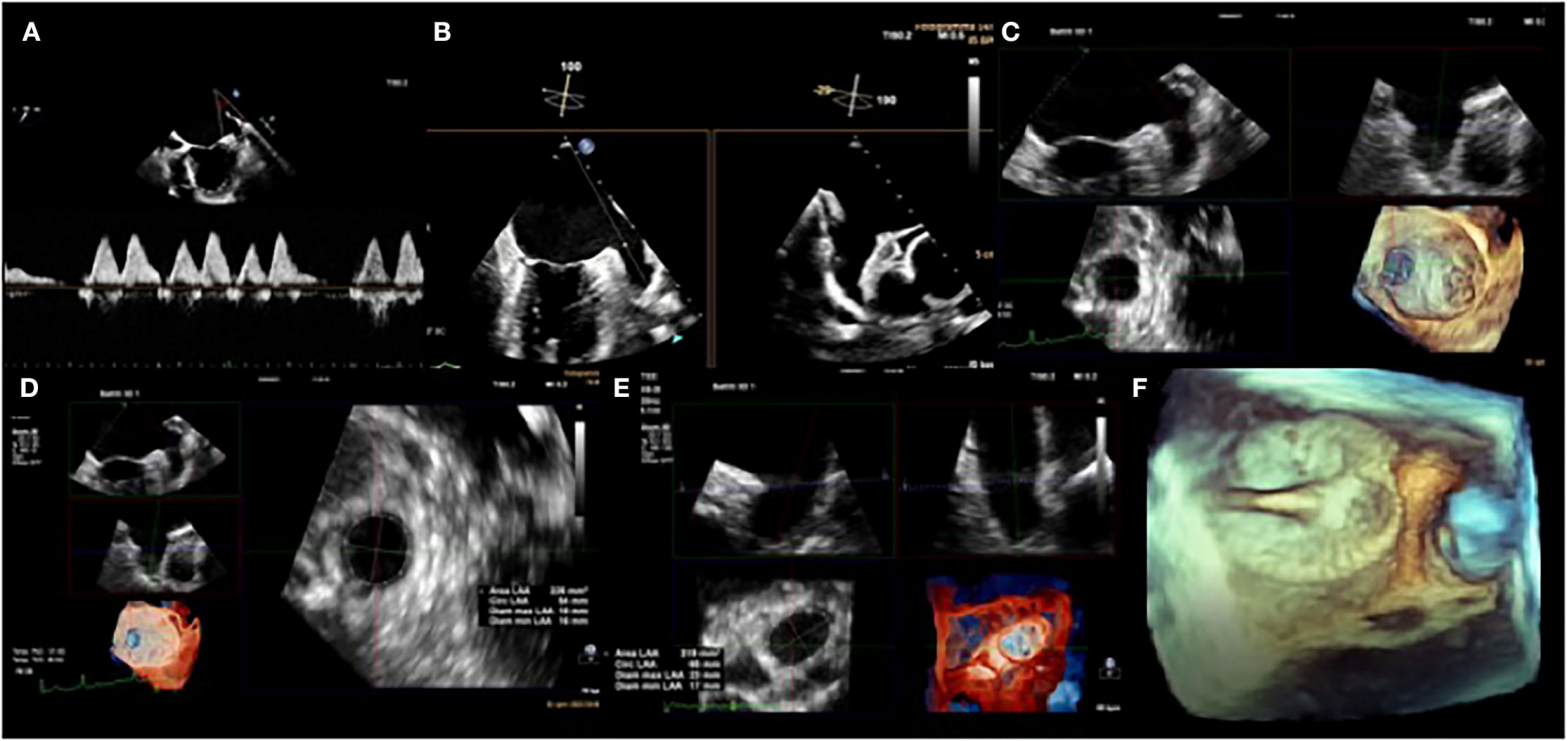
Transesophageal echocardiogram. Left atrial appendage (LAA) flow velocity pattern by pulsed-wave Doppler (A). 2D TEE biplane LAA assessment. Note how the appearance of the appendage varies in the different views (B). 3D TEE evaluation of the LAA landing zone (C), with post-processing analysis measurements of the diameters and the area of the LAA (D,E). 3D colorized depth map of LAA occluder device using peri-operative TEE (F).
